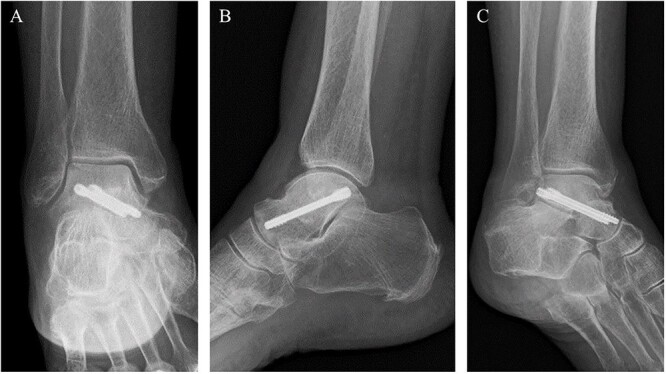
Post-operative plain radiographs revealed that two cannulated 4.5-mm double-threaded screws were inserted from the posterior part of the talus to the talar head as seen from the (A) anteroposterior, (B) lateral and (C) oblique views.
